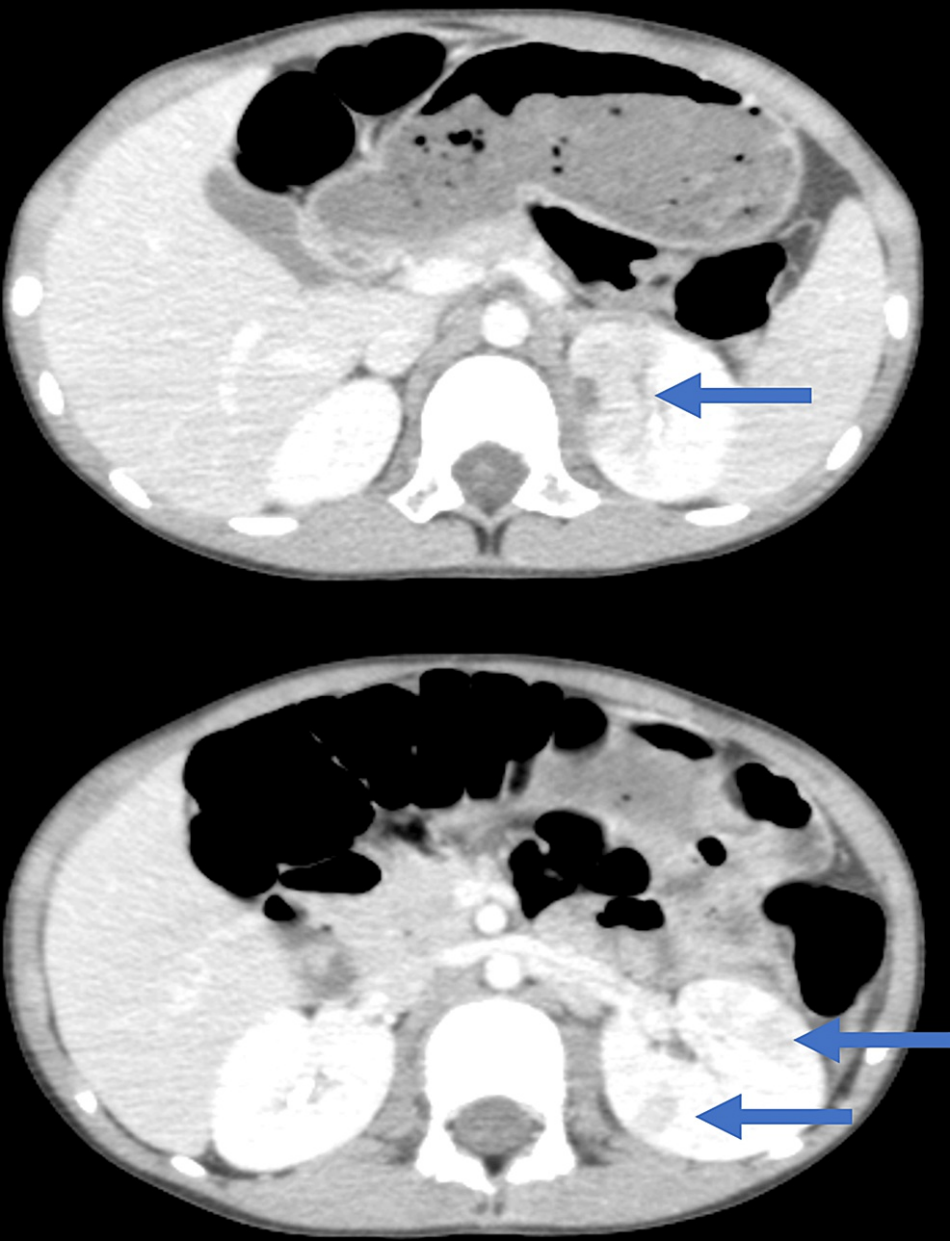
Contrast-enhanced abdominal computed tomography scans showing multiple low-density areas in the left kidney (arrow)
